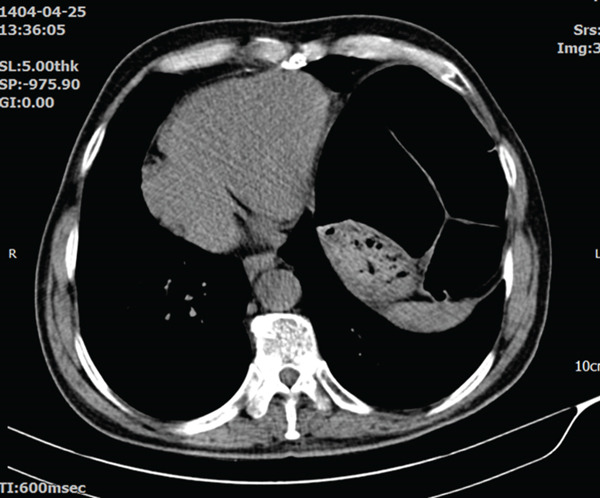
Chest CT scan (axial view). Congenital diaphragmatic eventration with intrathoracic herniation of dilated colon (megacolon) in the left hemithorax causing mediastinal shift.
